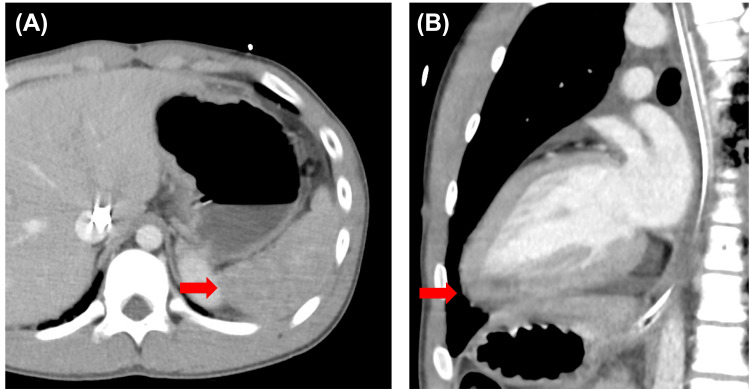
Contrast-enhanced computed tomography showing splenic ischemia and myocardial injury after a lightning strike Contrast-enhanced CT images demonstrating (A) splenic ischemia (arrow) and (B) poor contrast enhancement in the lateral and inferior walls of the left ventricle (arrow), suggesting myocardial injury likely caused by the lightning strike.
